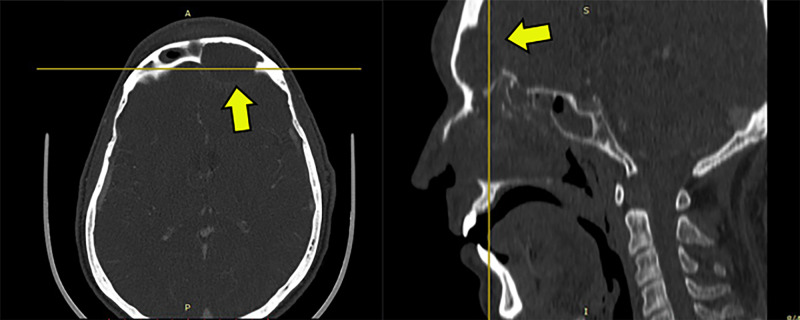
CT scan (Case 1) Contrast-enhanced axial computed tomography (CT) scan showed bony erosion of the internal wall of the frontal sinus as well as the existence of an abscess (yellow arrows).
